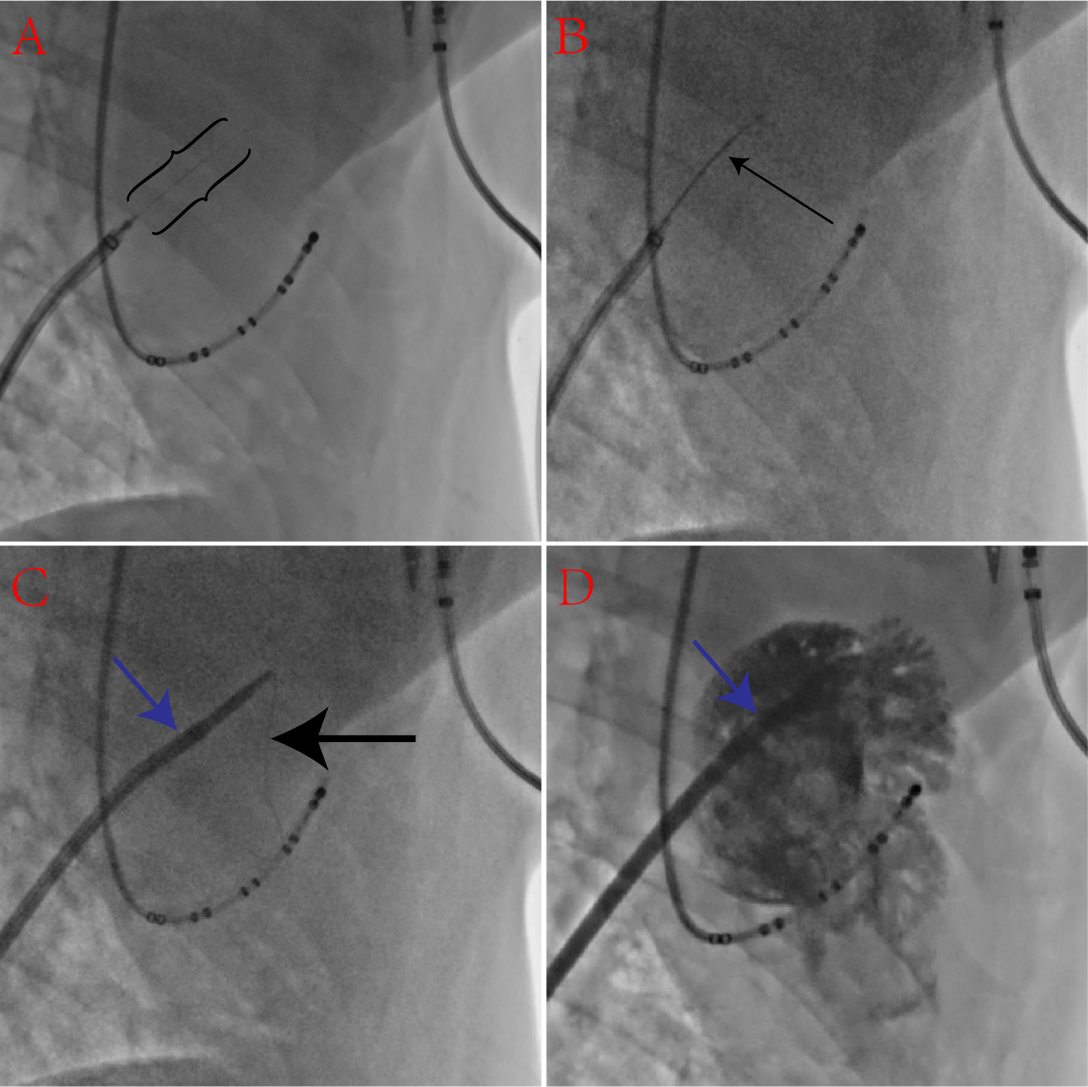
A sequence of X-ray images showing transseptal puncture with a
